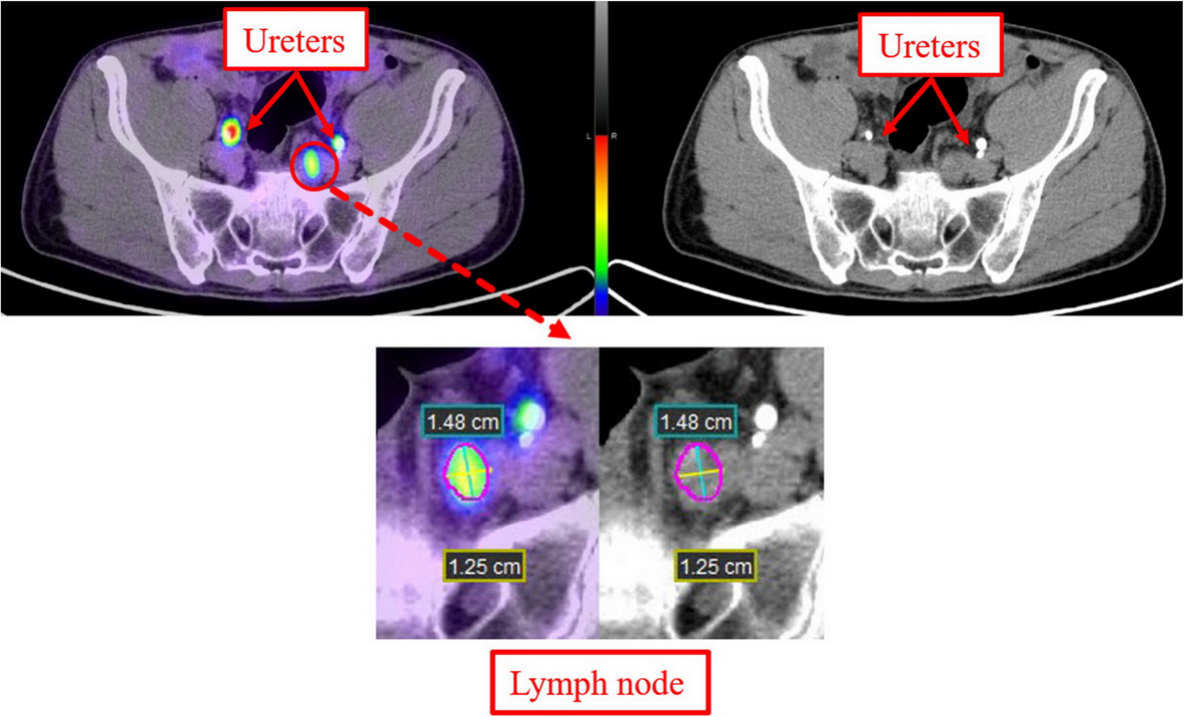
Top row. PET/CT-U and CT-U images show PSMA activity corresponding to opacified ureters and a left pelvic lymph node. The high-density focus posterior to the left ureter is a phlebolith in the iliac vessel. Bottom row. Magnified PET/CT-U and CT-U with semi-automatic PET edge detection with lymph node measurements
